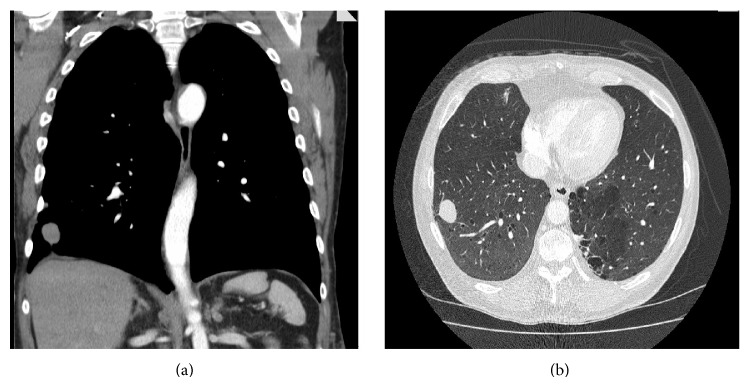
CT imaging of lung metastasis in coronal (a) and axial (b) planes.
